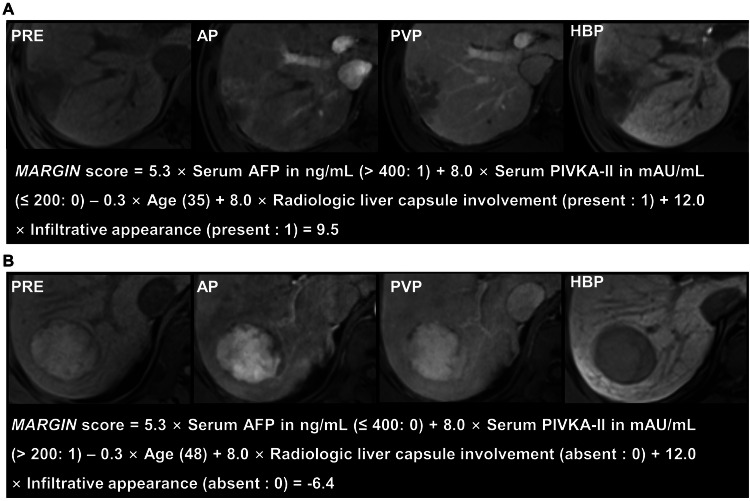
Contrast-enhanced MRIs of high-risk (A) and low-risk (B) patients with narrow resection margins. A A 35-year-old man with a 4.3 cm single HCC in segments VI and VII. Serum AFP was over 1210 ng/mL, and PIVKA-II was 141 mAU/mL. The tumor shows an infiltrative appearance and involvement of the liver capsule on MRI. This patient was classified as high-risk based on a *MARGIN* score of 9.5. The patient underwent a narrow resection margin and experienced recurrence at 2.1 months. B A 48-year-old man with a 5.2 cm HCC located in the segment VI and VII. The patient had a serum AFP level of 192 ng/mL and a PIVKA-II level of 1217 mAU/mL. The tumor does not show radiologic involvement of the liver capsule or infiltrative appearance. Based on a *MARGIN* score of − 6.4, the patient was categorized as low-risk. The patient received a narrow resection margin and remained recurrence-free during a 40.2-month follow-up. HCC, hepatocellular carcinoma; AFP, alpha-fetoprotein; PIVKA-II, protein induced by vitamin K absence or antagonist-II; PRE, pre-contrast phase; AP, arterial phase; PVP, peritumoral portal venous phase; HBP, hepatobiliary phase
